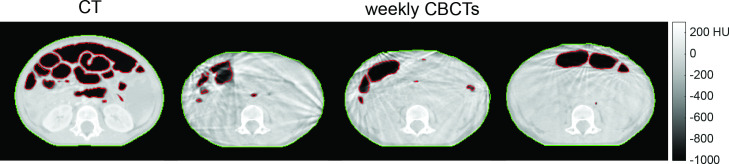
Example of variability in gastrointestinal gas and body contour between planning computed tomography (CT) and multiple weekly cone beam CT (CBCT) scans (Gas_std_ = 171 ml for this subject).
